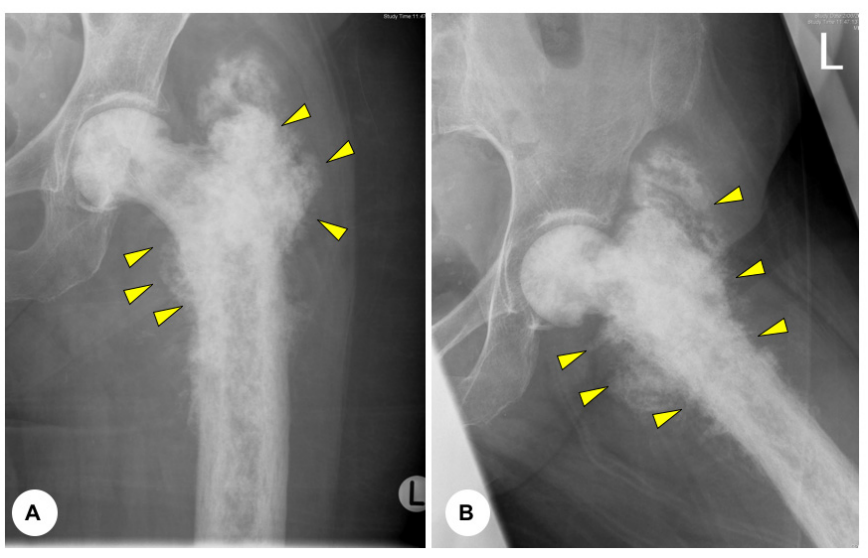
(A) and (B) Anteroposterior and lateral plain radiographs displaying typical pagetoid changes to the proximal femur with evidence of tumour extending beyond the cortex into the soft tissue (arrows). This 85 year old lady was managed conservative with radiotherapy alone.
